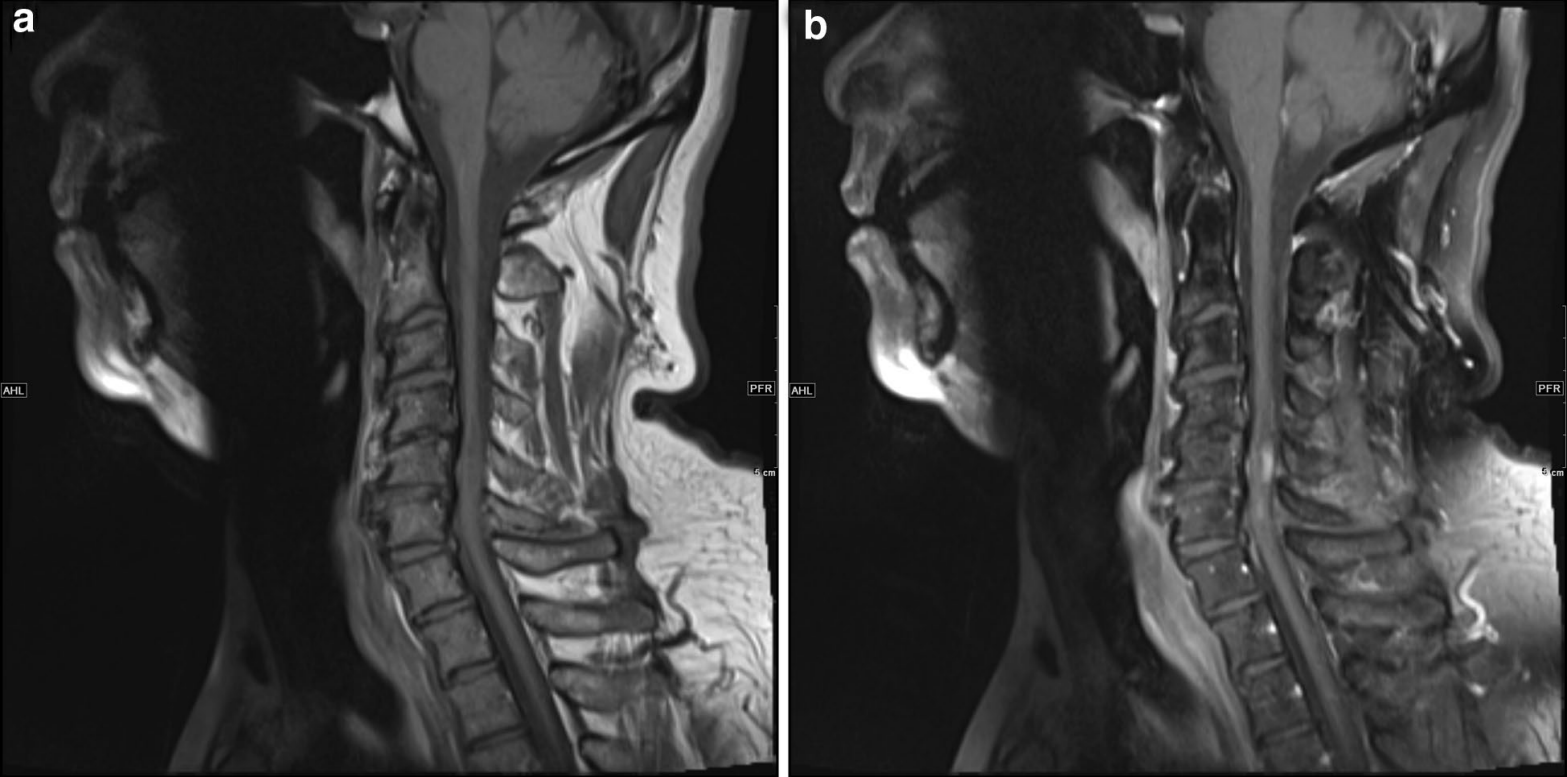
Sagittal T1 MRI (a) of the cervical spinal cord shows an area of mixed signal intensity as well as slight expansion at C4–C5 and C5–C6. Post-contrast images (b) show an area of enhancement within the cord at the level of C4–C5.
